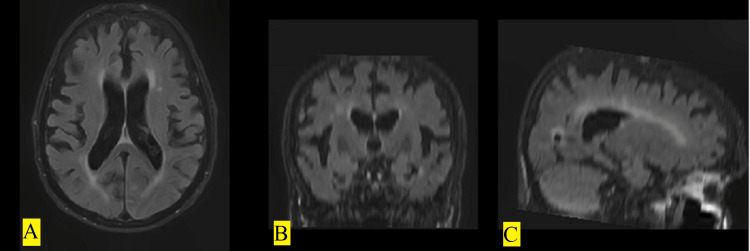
Magnetic resonance images in FLAIR sequence in (A) transverse, (B) coronal, and (C) sagittal planes. FLAIR: fluid-attenuated inversion recovery
